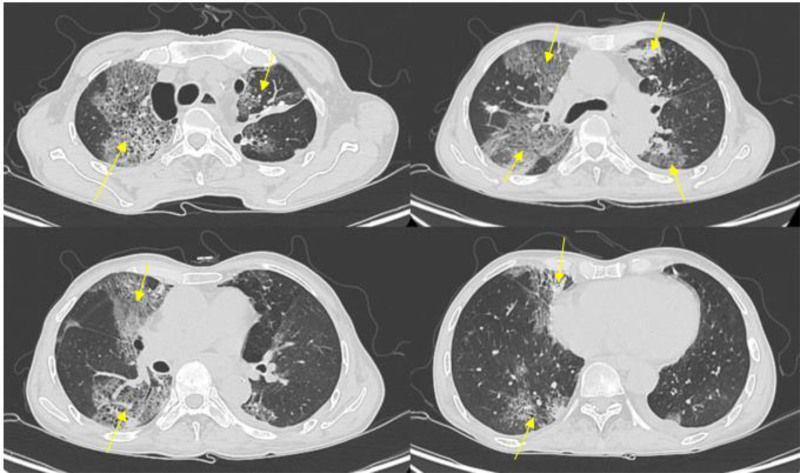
Initial chest CT scan: honeycomb-like triangular mixed alveolar-interstitial opacification in both lungs (thin yellow arrow).
